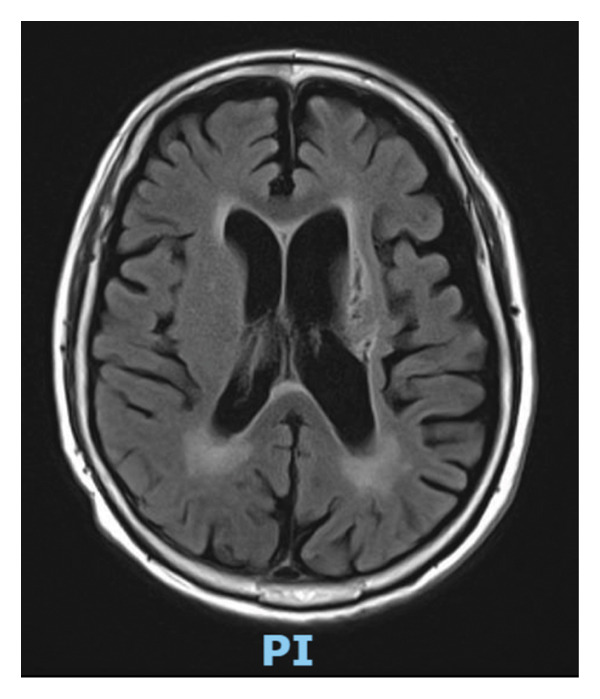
T2‐FLAIR of a magnetic resonance imaging (MRI) scan of the brain with reported old infarcts in the left striatocapsular region, right lentiform nucleus, right hemipons, and right cerebellum.
